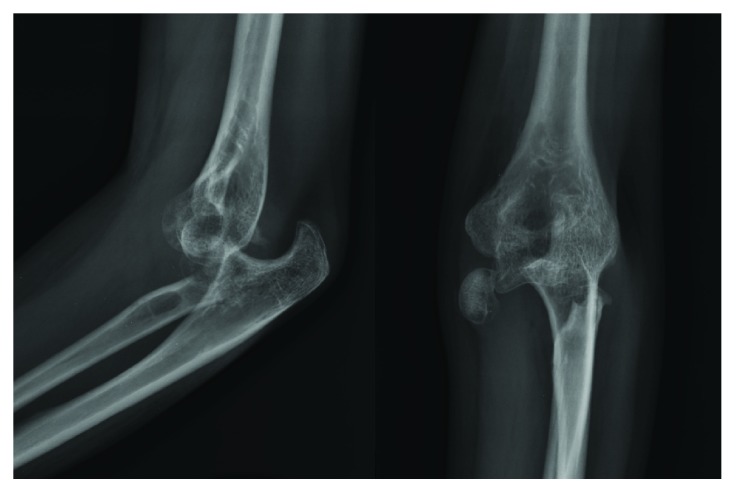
Plain radiographs showing posterior elbow dislocation with displaced radial head.
